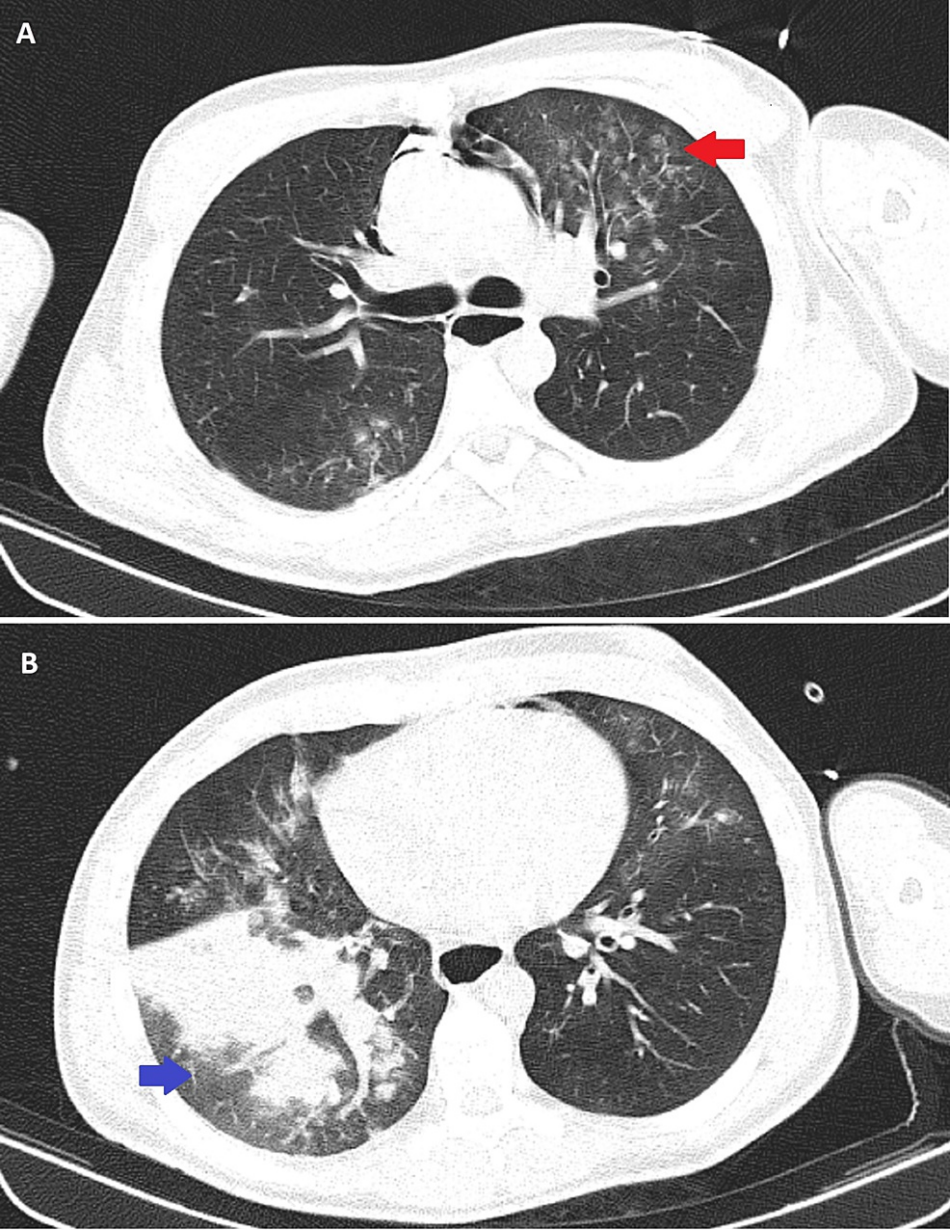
Chest computed tomography showing: (A) pulmonary infiltration in the left superior lobe (red arrow); (B) a consolidation in the right inferior lobe (blue arrow).
